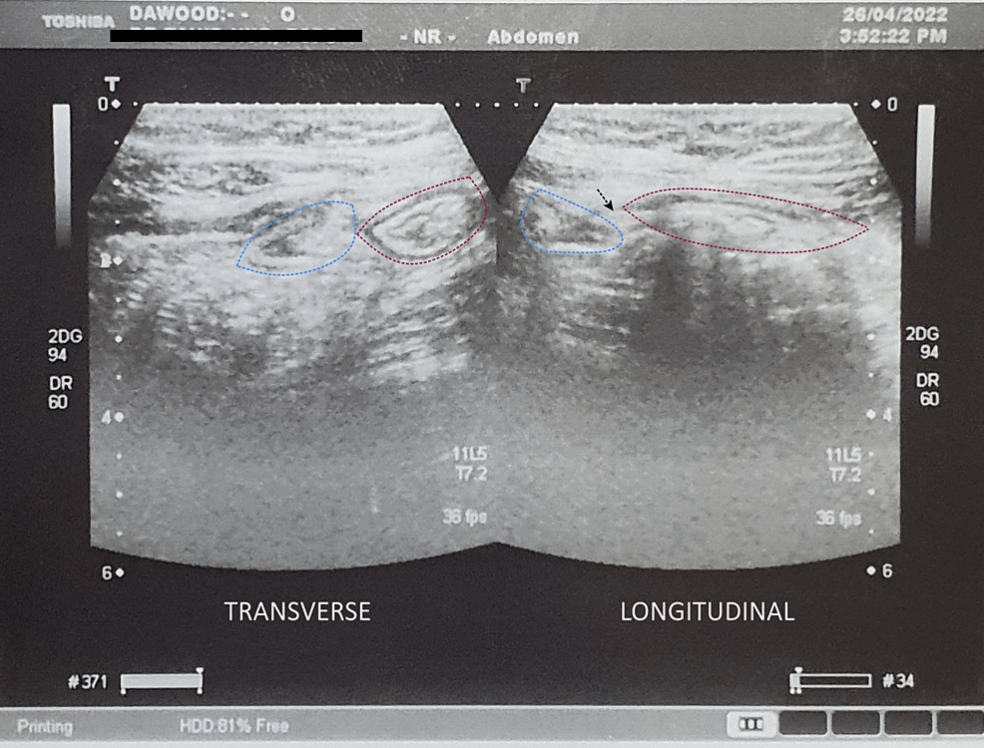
Abdominal ultrasound performed following a three-day course of intravenous antibiotics showing significant improvement. The terminal ileum is now mildly edematous and is encased by a thin streak of fluid. The appendicular stump, measuring 10 mm, is encased by a small focus of thickened mesentery. Dotted red line, terminal ileum; dotted blue line, appendicular stump; black arrow, thickened mesentery.
